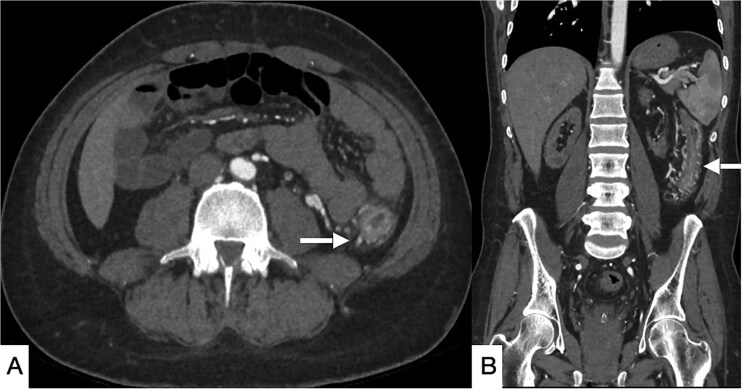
Selected CT angiogram of the abdomen and pelvis in axial (A) and coronal (B) planes showing mild diffuse thickening and mucosal hyper-enhancement of the descending colon, with prominent feeding mesenteric arteries and early draining vein dilation, consistent with angiodysplasia.
